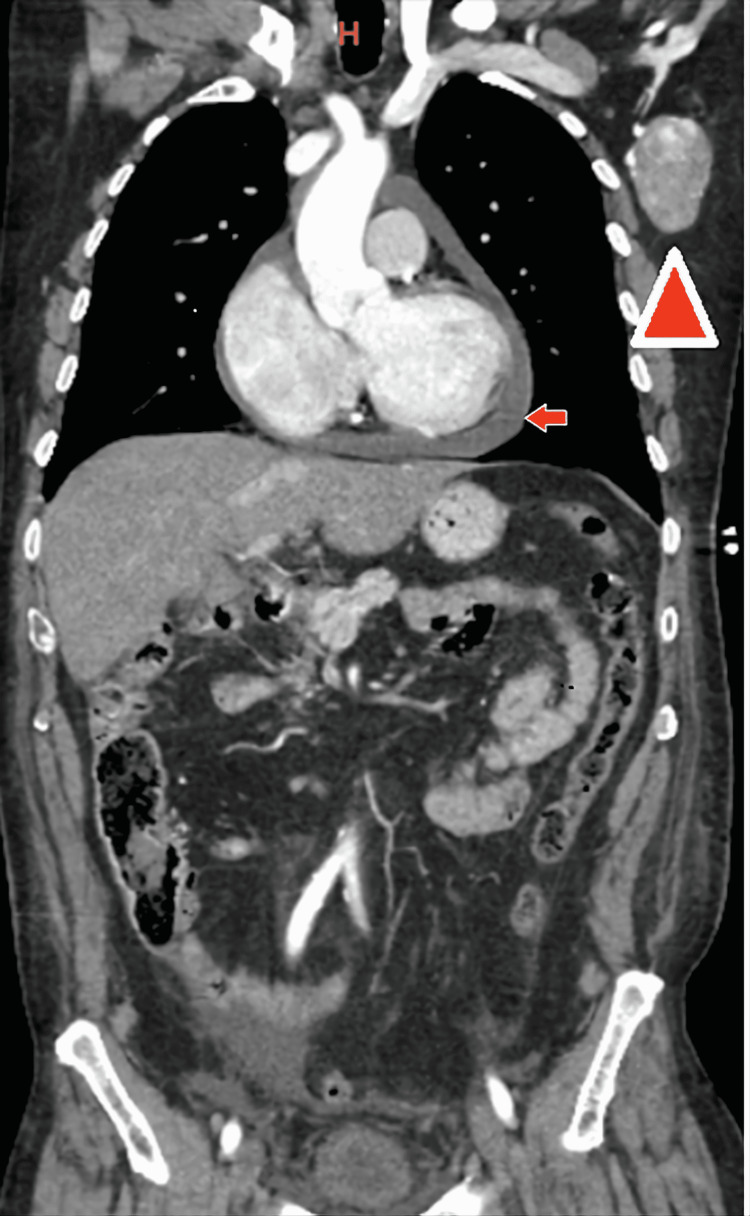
CT of chest, abdomen, and pelvis (coronal view) revealing large left lymphadenopathy (arrowhead) and pericardial effusion (small arrow)
